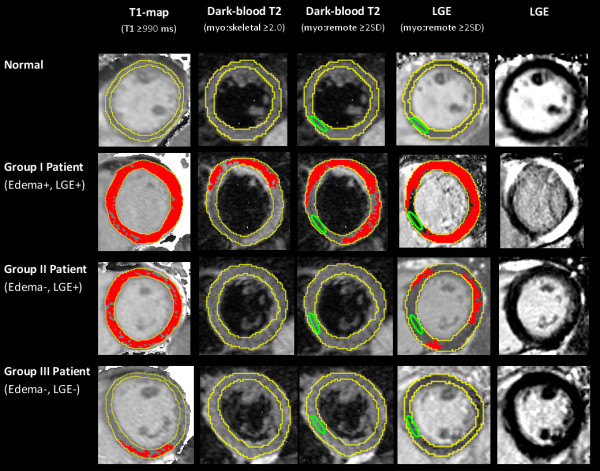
T1-maps detected the location and extent of myocardial injury in acute myocarditis. Areas of myocardial injury (red) as detected by computer-aided analysis of T1-maps, T2-weighted and late gadolinium enhanced (LGE) images in a normal subject (row 1) and patients with acute myocarditis (rows 2–4). Red indicates areas of myocardium with values above the stated threshold for each method. Green contour marks the position of reference region of interest (ROI). Skeletal muscle ROI for T2W images not shown. Note that a T1 cut-off of ≥ 990 ms detected larger areas of injury than corresponding T2W and LGE imaging analysis.
